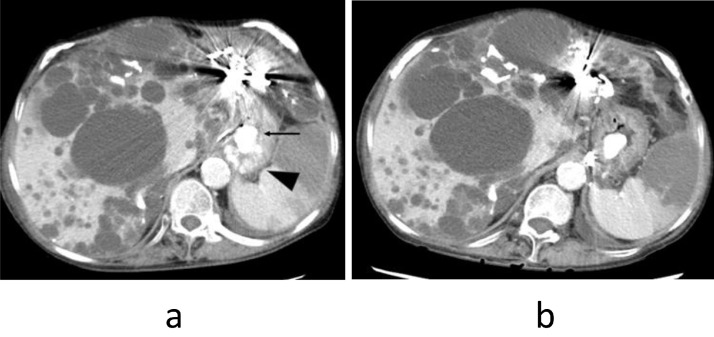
(a). Contrast-enhanced CT after the emergency hybrid procedure in case 2 shows NBCA-Lipiodol (arrow), residual gastric varices (arrowhead), and partially infarcted spleen. (b). Contrast-enhanced CT after TJO shows obliterated residual gastric varices.
